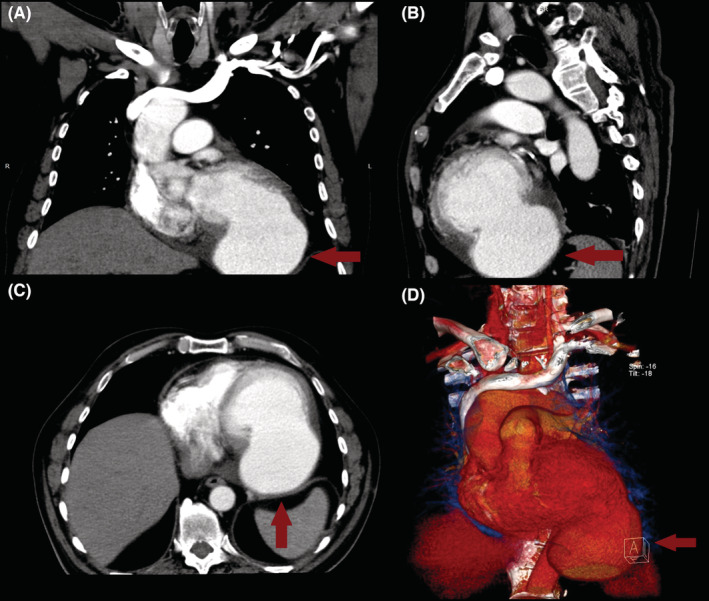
Images of chest computed tomography; (A) frontal view, (B) sagittal view, (C) axial view, (D) 3D volume rendering confirm the diagnosis of giant left ventricular pseudoaneurysm
